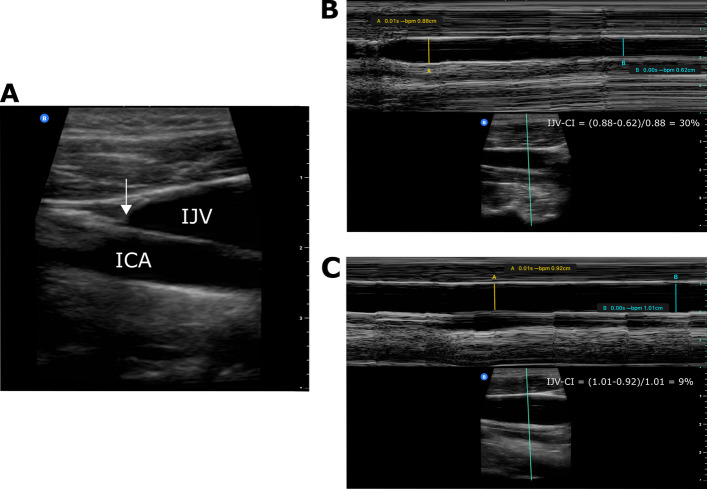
Point-of-care ultrasound (POCUS) of the internal jugular vein (IJV). A A typical POCUS image depicting the IJV, internal carotid artery (ICA), and IJV collapse point (arrow). B IJV POCUS suggesting the absence of volume overload with IJV collapsibility index (IJV-CI) ≥ 30%. C IJV POCUS suggesting the presence of volume overload with IJV-CI < 30%
